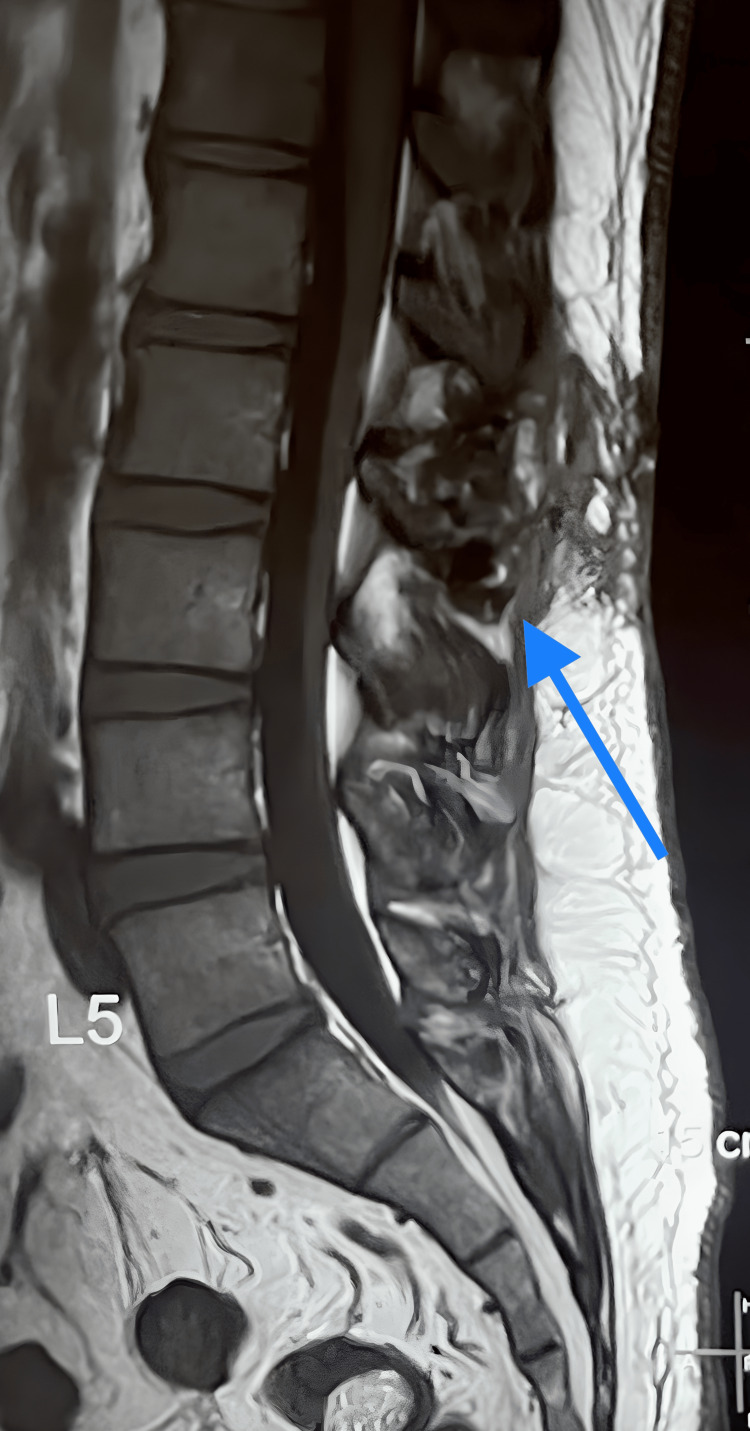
Follow-up T1 weighted MRI sagittal view Postoperative 18-month follow-up T1 weighted MRI image, arrowhead showing scarred soft region without any spinal cord structural abnormalities
